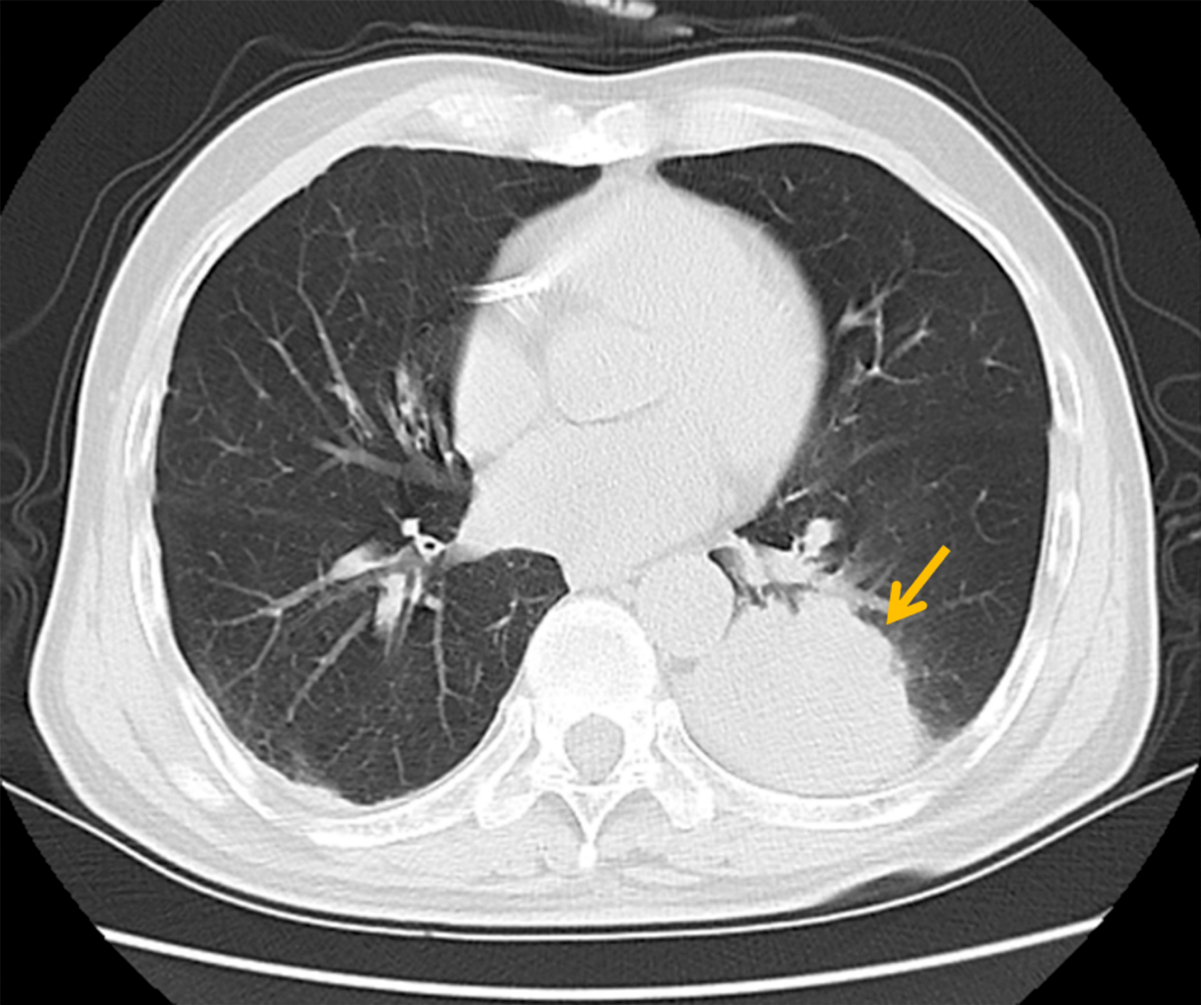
Chest CT showing a 6-cm mass in the left lower lobe (arrow)
